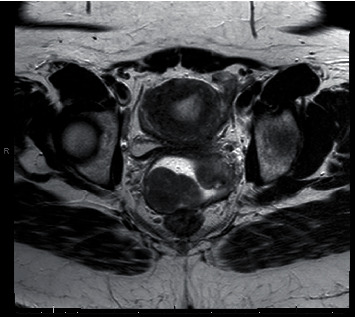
Axial T2 MRI with separate cervix (patient left) and vaginal mass (patient right).
